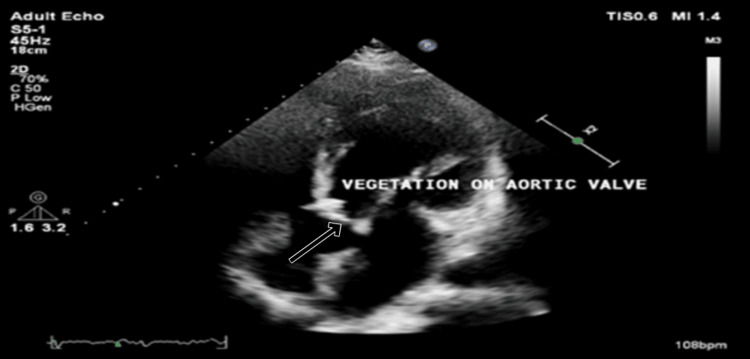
Apical 5-chamber (A5C) view on 2D echocardiography demonstrating vegetation on the aortic valve (arrow).
